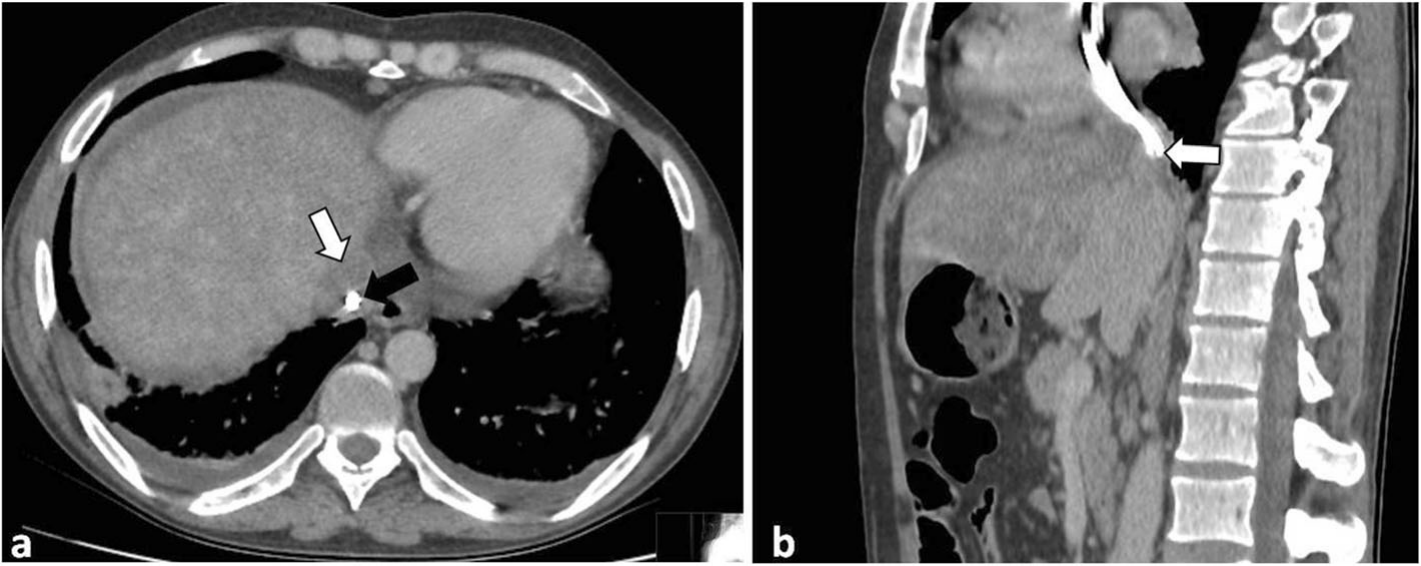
a and b In axial contrast venous phase CT section (a), the distal end of the dialysis catheter is located at the level of drainage of the hepatic veins in the inferior vena cava (black arrow) and the hypodense thrombus (black arrow) around the catheter that prevents hepatic vein drainage. In the sagittal reformat CT image, the distal of the catheter located vena cava inferior is also observed (white arrow, b)
